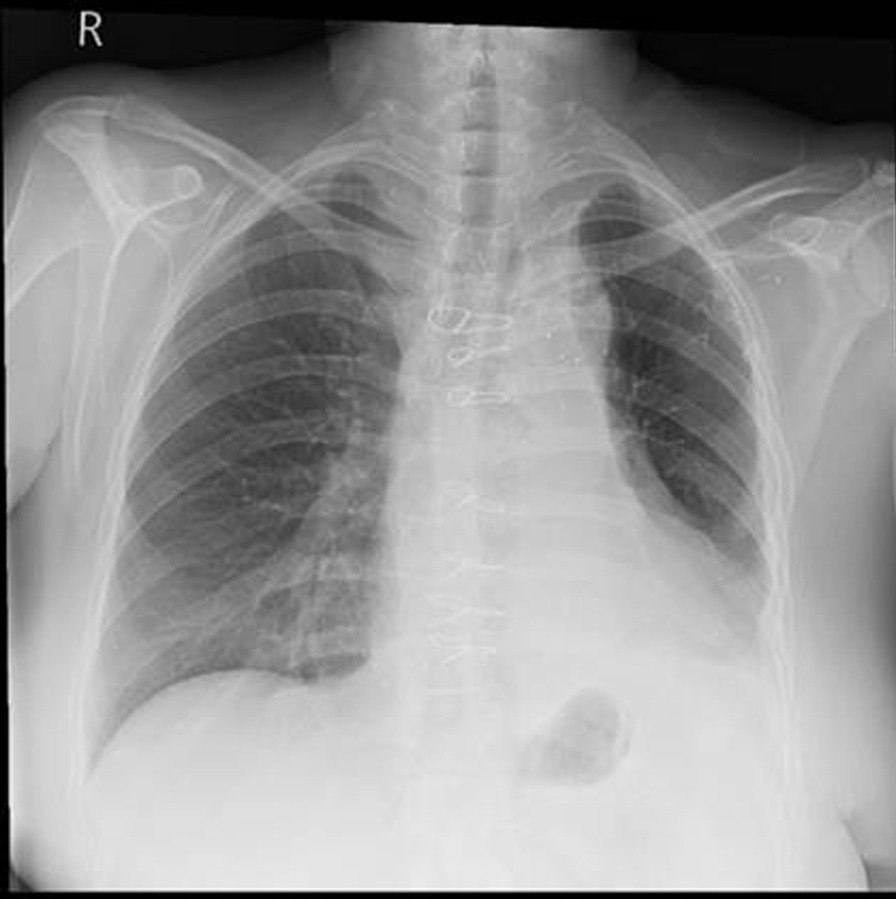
Chest x-ray prior to hospital discharge from July 2022, resolution of left upper lobe collapse
